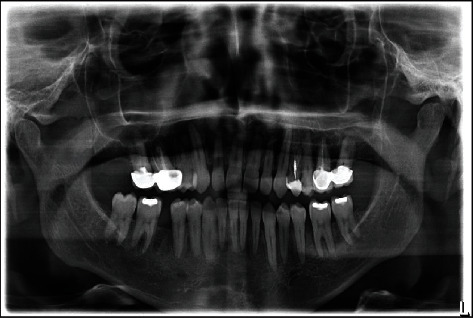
Pretreatment panoramic radiograph of initial patient presentation.
